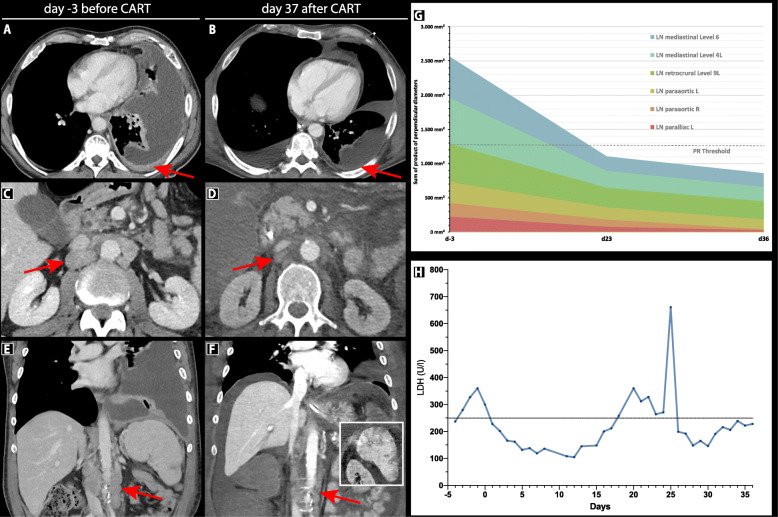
Radiographic evidence of partial response to Axi-cel after 36 days. Contrast-enhanced CT abdomen (axial view) displaying pleural manifestations before (a) and after (b) CAR T-cell treatment. Involved paraaortic lymph nodes (red arrows) demonstrate an interval decrease in size (c-d, e-f). The inset of panel F depicts splenic hypodensities consistent with necrosis of tumor tissue secondary to immune therapy and a reduction of splenomegaly. G Graphical depiction of response over time. Tumor extent was quantified as the sum of the product of perpendicular diameters according to Lugano criteria over 3 time points and is graphed on the y-axis. H Timeline of LDH levels (U/l). The increase in LDH levels at day 25 correlated with the gastrointestinal toxicity and liver dysfunction
